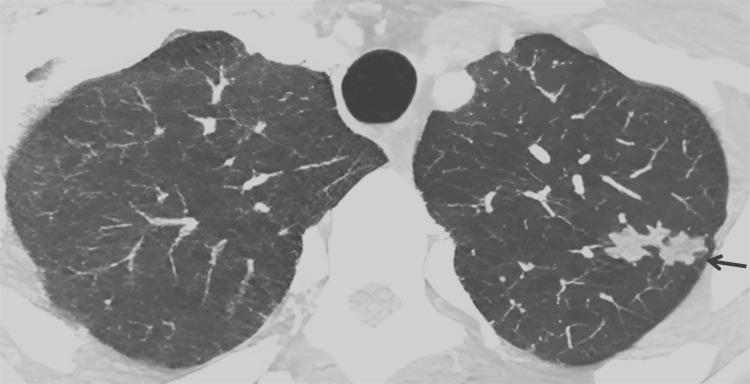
Enhanced axial slice of chest CT in the lung window with the maximum intensity projection (MIP) showing a tree-in-bud appearance in the left upper lobe (black arrow), highly suggestive of endobronchial dissemination of infectious diseases.
